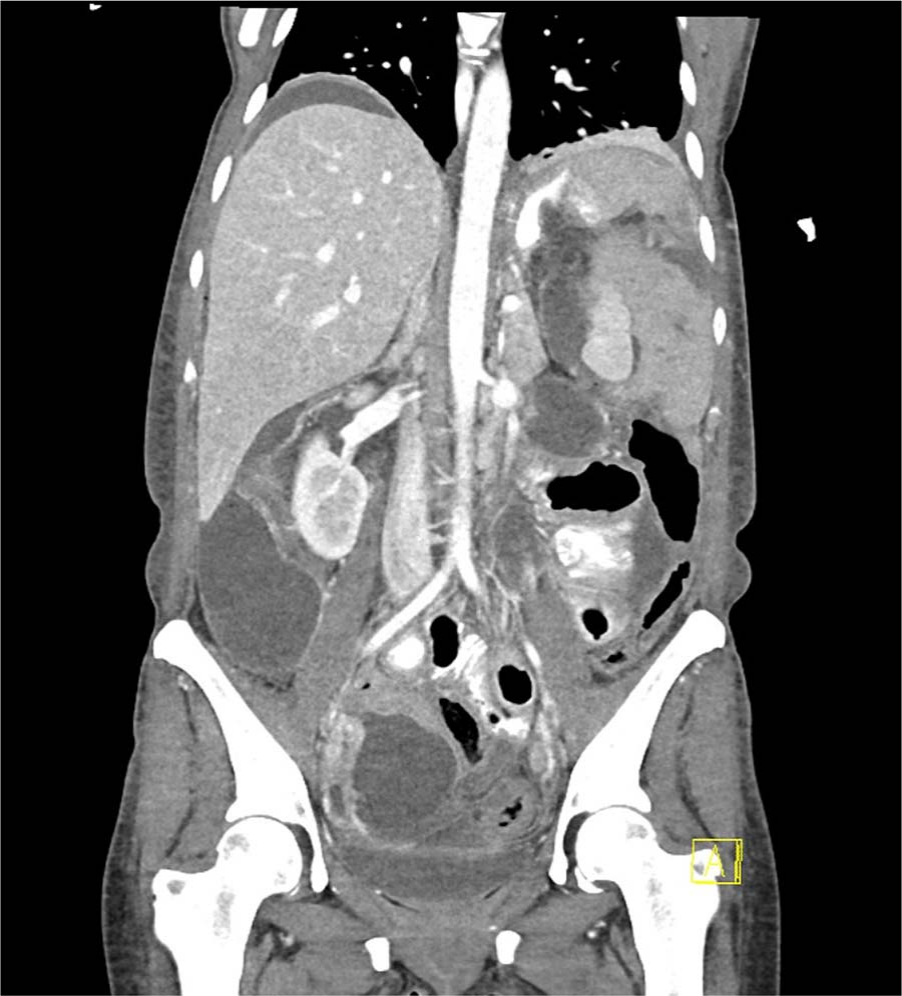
Coronal portal venous phase CT scan with oral contrast of the abdomen and pelvis demonstrating the proximal gastric leak with large volume contrast extravasation into the left upper quadrant and widespread intra-abdominal fluid with pneumoperitoneum.
